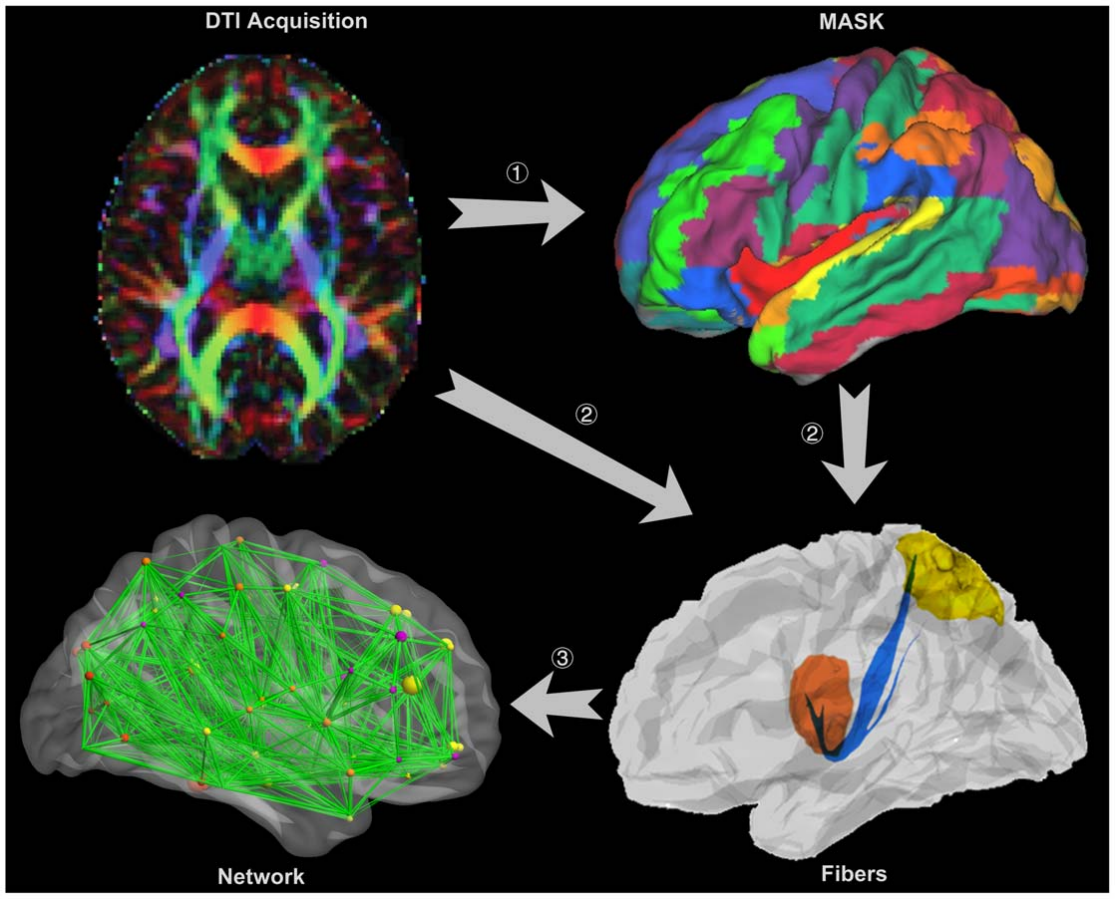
Extraction of a whole brain anatomical network. The DTI image is presented in a reconstructed color-coded tensor map, showing the direction of the principal axis of diffusion using the standard scheme. Blue codes for the superior-inferior, red for left-right, and green for anterior-posterior orientation. (1) Cortical parcellation. The DTI images are mapped with an AAL atlas in the diffusion-MRI native space. (2) Fiber tractography between ROIs. Probabilistic tractography is performed between two ROIs defined in step (1), with only direct connections being retained. (3) Whole brain anatomical network construction. All of the connections in step (2) constitute the whole brain anatomical network.
